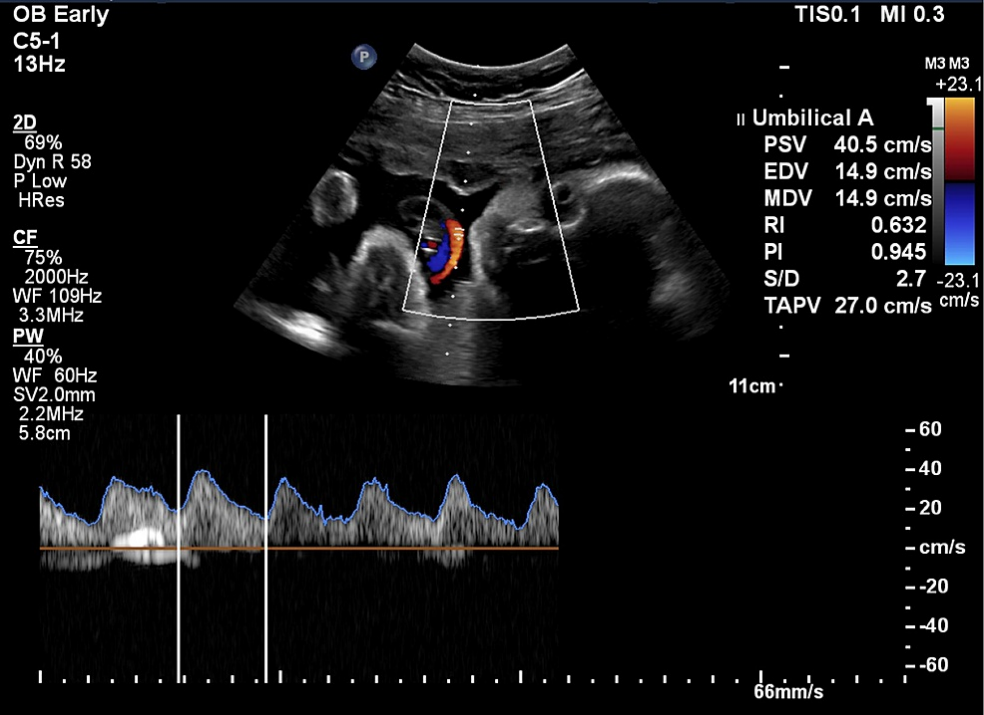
Umbilical artery Doppler ultrasound for a singleton pregnancy at 28 weeks gestation reveals normal values.
